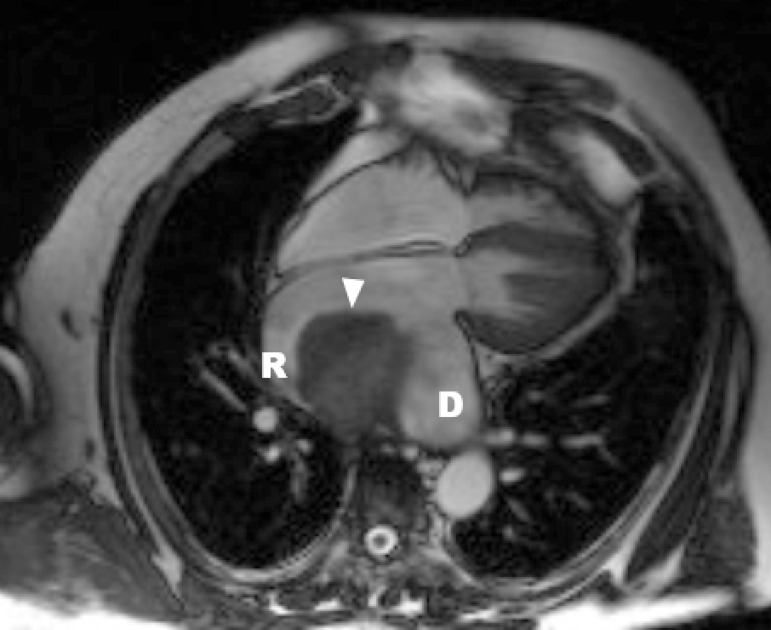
Cross-sectional magnetic resonance imaging of the thorax. Four-chamber projection. Giant intracardiac thrombus (arrow) whose base is attached to left atrial wall in suture lines between the recipient’s (R) left atrium and the donor’s (D) left atrium.
